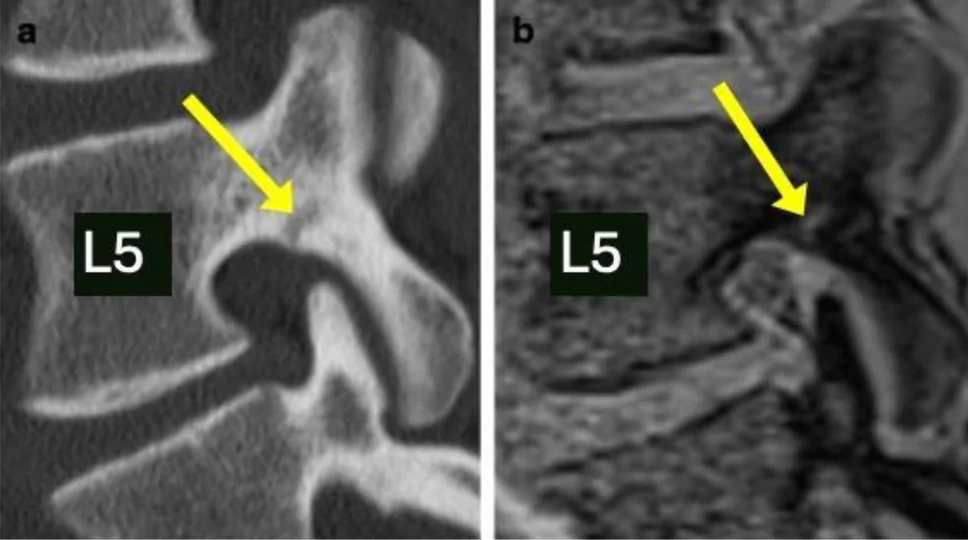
(a) Sagittal CT of L5 and (b) sagittal 3-T MRI T-1 VIBE (volumetric interpolated breath-hold examination) sequence both demonstrating pars fracture at L5 (fracture indicated by yellow arrows) [39]; reproduced from Ang et al. [39], with permission
